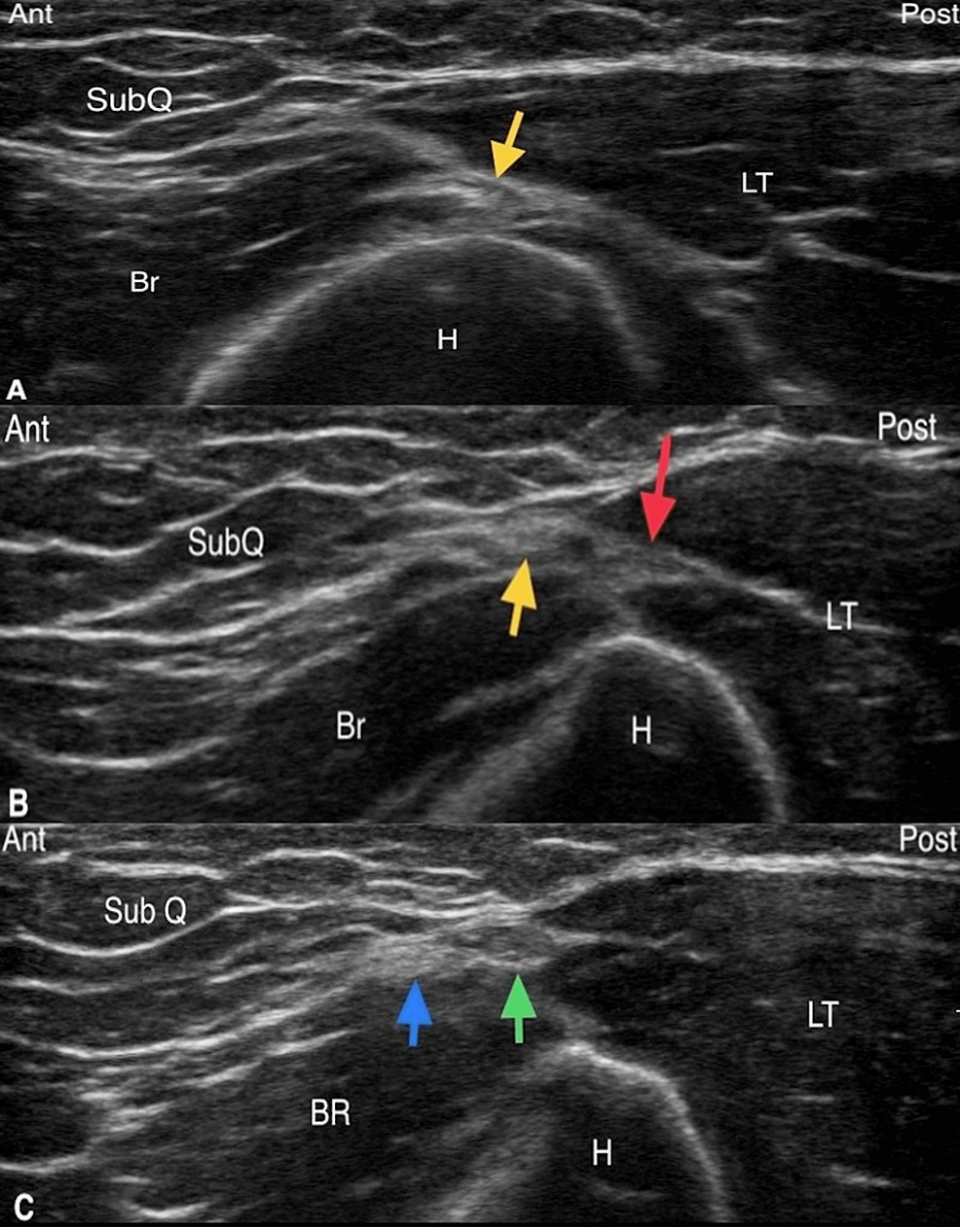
(A) Sonographic identification, in the transverse axis view, of the radial nerve (yellow arrow) at the level of the spiral groove at the lateral posterolateral humerus. (B) Transverse axis view depicting the PCNF (red arrow) arising as the posterior division of the radial nerve (yellow arrow) upon exiting the spiral groove. (C) By the distal third of the humerus, the transverse view at the lateral brachium shows the PCNF dividing into an anterior division (blue arrow) and a posterior division (green arrow) within the LT-BR interval. SubQ: subcutaneous tissue; Br: brachialis; LT: long head of the triceps; H: humerus; BR: brachioradialis; Ant: anterior; Post: posterior; PCNF: posterior cutaneous nerve of the forearm; LT-BR: lateral triceps-brachioradialis
